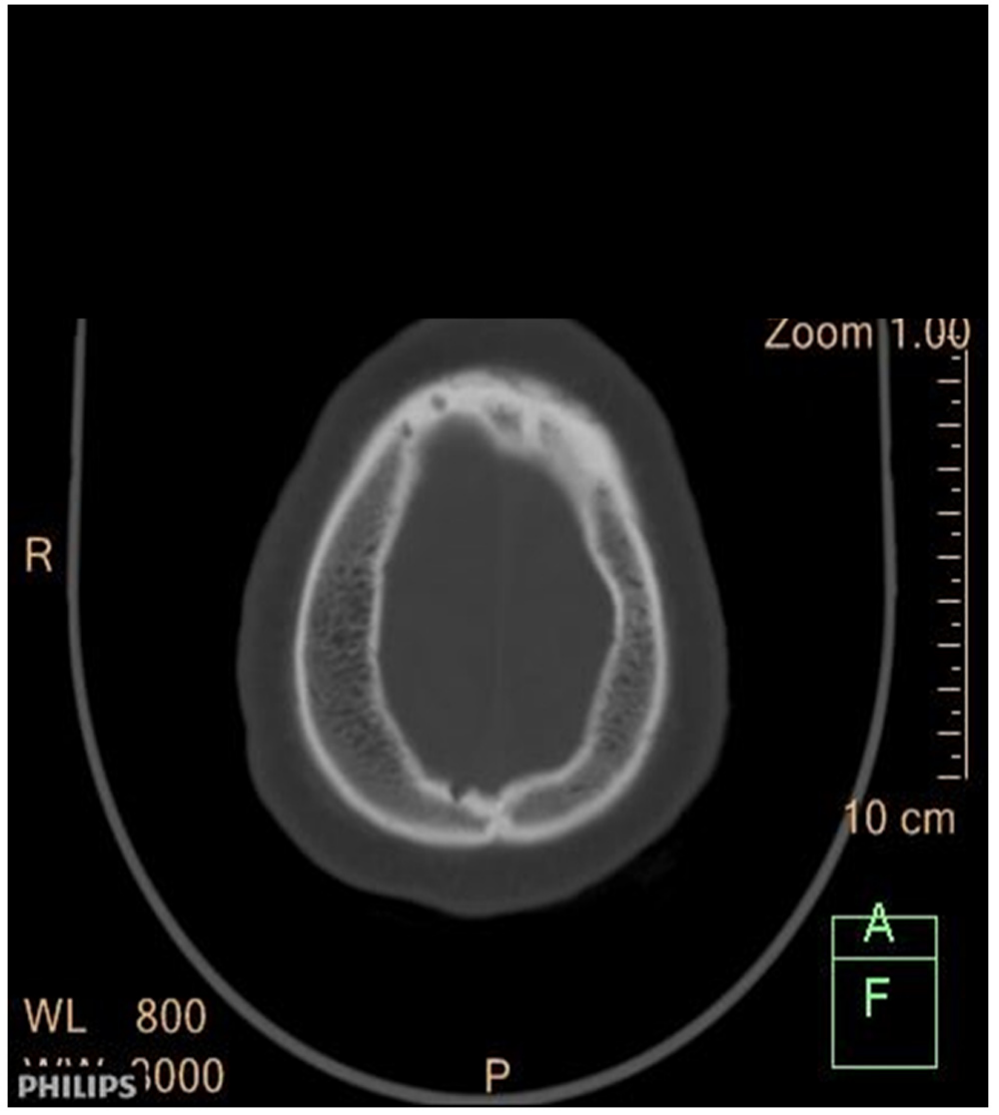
CT scan of the head (axial view). Shows a marked thickness on the right side of the skull.
